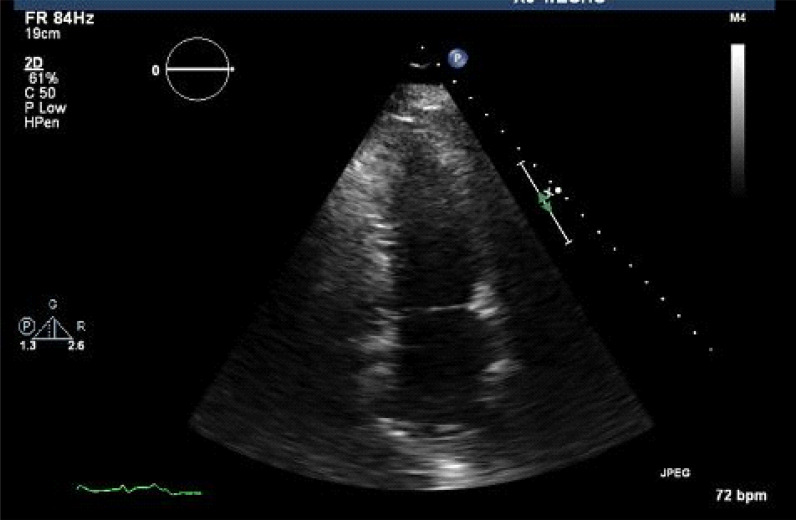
Echocardiography showed normal left ventricular function with no wall-motion abnormality.
